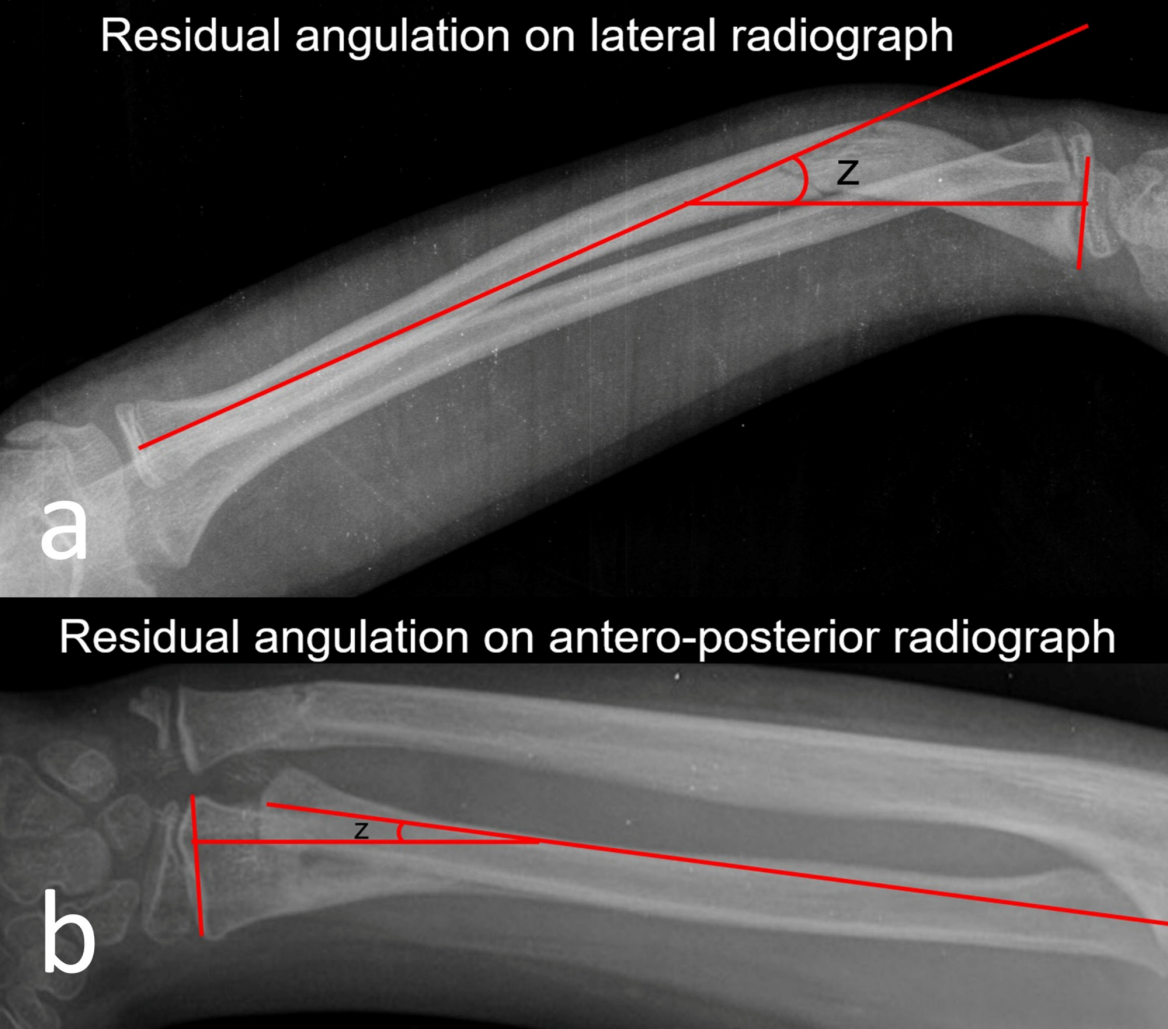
References about measurement of residual angulations. (a) Measurement of residual angulation on lateral radiograph, and (b) residual angulation on anteroposterior radiograph.
